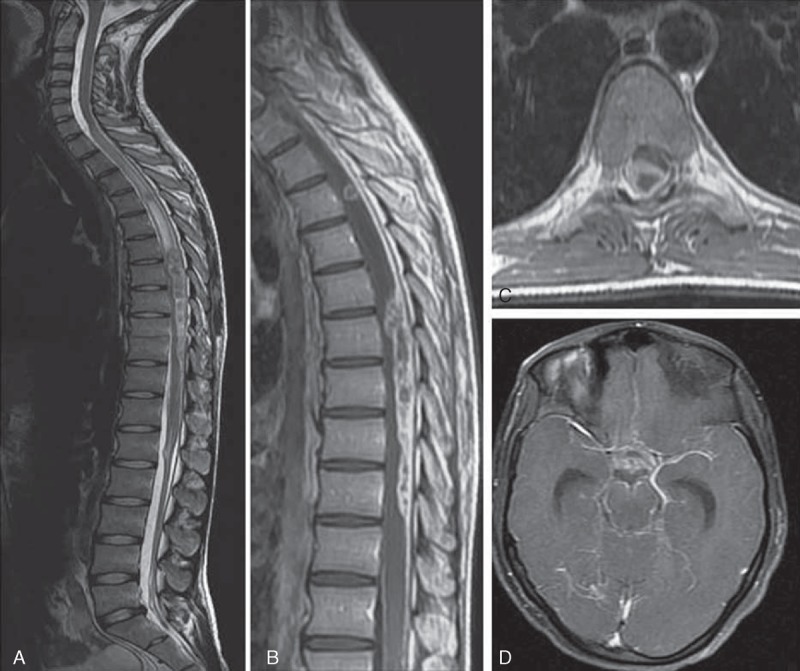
MRI spine of a 22-year-old man with tuberculous meningitis, the patient presented with paraparesis, of 4 weeks duration. T2-weighted image shows long segment myelitis extending from D3 to D6 thoracic level (A); a rounded intramedullary hypointensity at D4 level showing ring enhancement in postcontrast fat suppression imaging (B) and intradural–extramedullary collection extending from D6 to D10 level (A–C). Also, there is meningeal enhancement surrounding the conus medullaris (B). MRI brain of the same patient revealed basal meningeal enhancement and hydrocephalus (D). MRI = magnetic resonance imaging.
